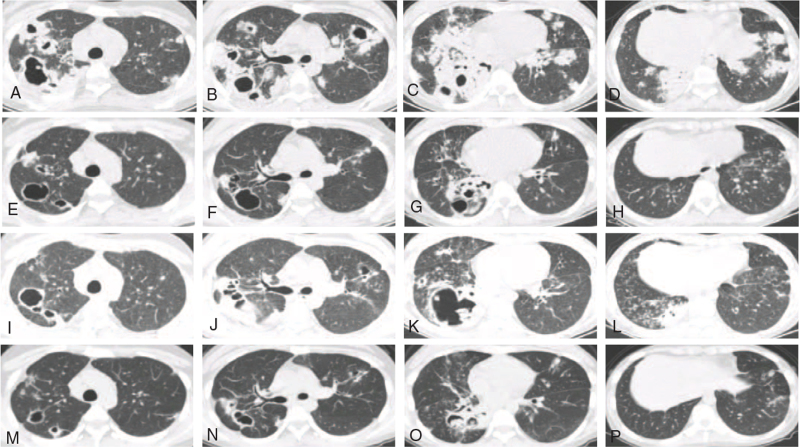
Follow-up chest computed tomography scans. A–D: Onset, E–H: After *C neoformans* target treatment (6 months ago), I–L: Recurrence pulmonary infection, M–P: After *Aspergillus* target treatment (3 months ago).
